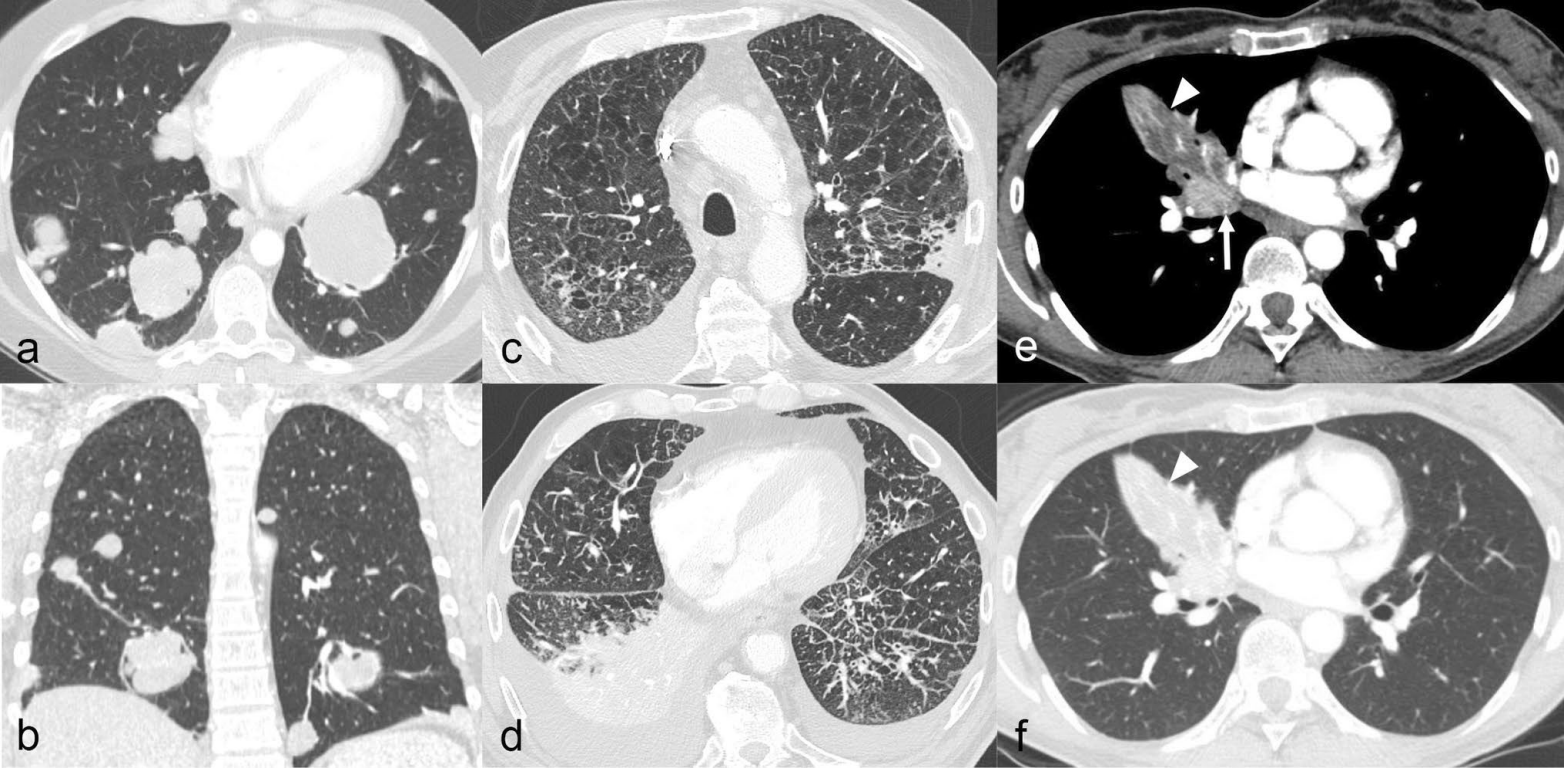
Typical CT appearance and distribution of hematogenous pulmonary metastases, lymphangitic carcinomatosis and endobronchial metastasis. a, b Lung metastases in a 32 y.o. woman affected by Ewing sarcoma. Axial (a) and coronal (b) computed tomography (CT) images show multiple solid nodular and rounded lesions of variable size, preferentially located in the basal regions of the lungs. c, d Lymphangitic carcinomatosis in a 74 y.o. man affected by advanced stage colon cancer. Axial CT images show bilateral smooth thickening of the interlobular septa and peribronchovascular interstitium, predominant in the lung bases, associated with bilateral pleural effusion and atelectasis of the right lower lobe. Note the absence of architectural distortion of the secondary pulmonary lobules. e, f Endobronchial metastasis in a 57 y.o. patient with melanoma (e, f). Contrast-enhanced CT images show a well-defined, homogeneous and strongly enhancing solid nodule (white arrows in e), that obstructs the right middle lobe bronchus, determining distal lobar atelectasis (arrowheads in e, f)
